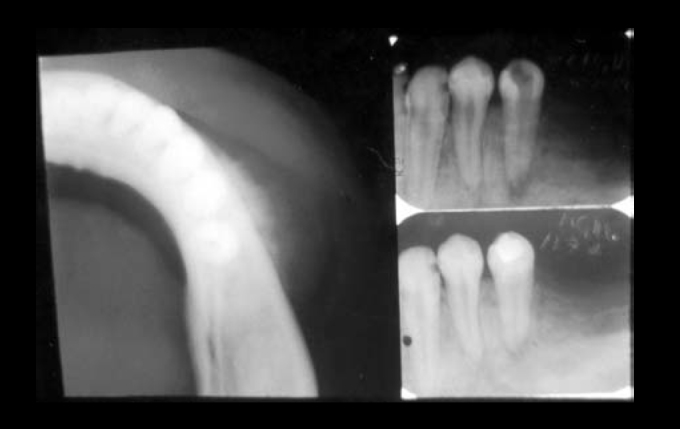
Occlusal radiographic view showing the external cortical portion with radiopacity similar to “sunrays” (on the left). Periapical X-rays showing increased periapical space of the premolar tooth (on the right).
